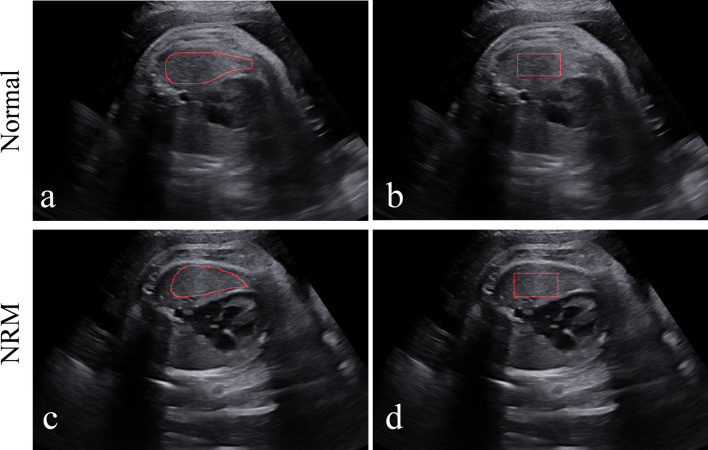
Examples of the lung region delineations in the lung ultrasound images of a normal fetus and a fetus with NRM. a and b are the irregular and square ROI selection in the ultrasound image of a normal fetus. c and d are the irregular and square ROI selection in the ultrasound image of a fetus with NRM
